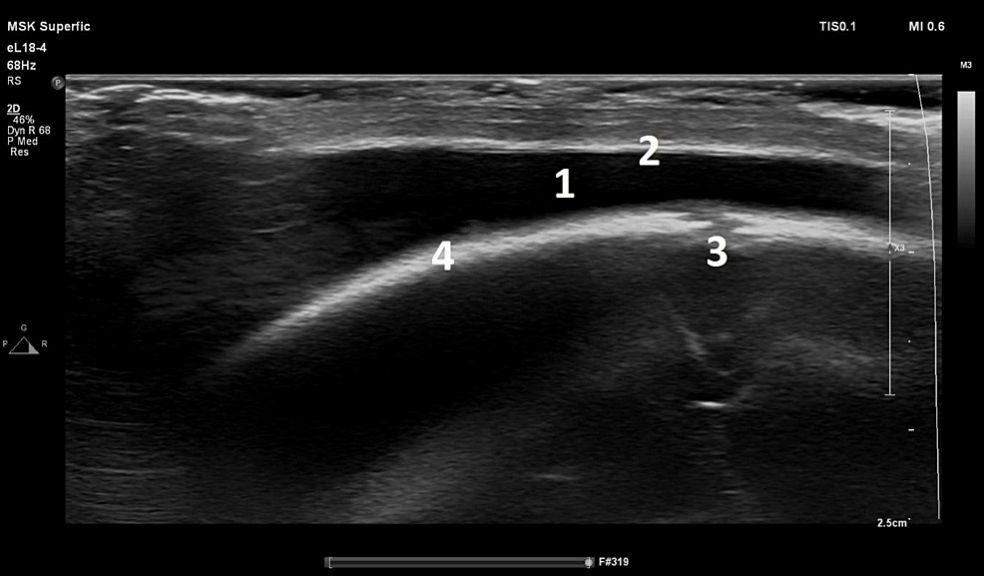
Cranial ultrasonography images Cranial ultrasonography image in coronal orientation done on admission of the case. (1) Subaponeurotic fluid collection with cerebrospinal fluid (CSF)-like hypodensity. (2) Galea aponeorotica layer of the occipitofrontalis muscle appearing as a thin hyperdense scalp layer. (3) The isodense irregular fibrous sagittal suture line marking the midline of the skull between the two pariteal bones. (4) The right parietal bone as a hyperdense structure following the curvature of the skull clavaria.
